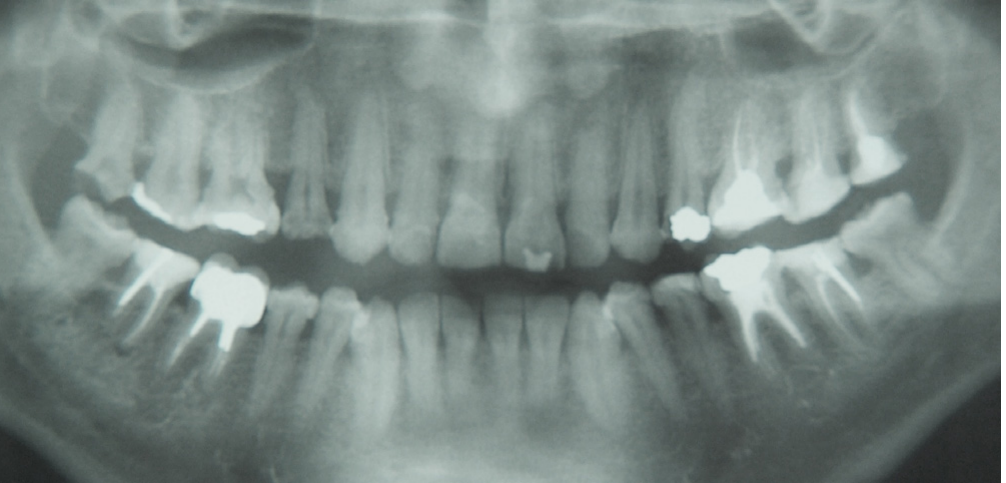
Patient 1: Panoramic radiograph at 36 years of age. The examination shows obliteration of the pulp chambers and reduced level of alveolar bone. The patient has a complete permanent dentition whereas he lost all this primary teeth prematurely.
